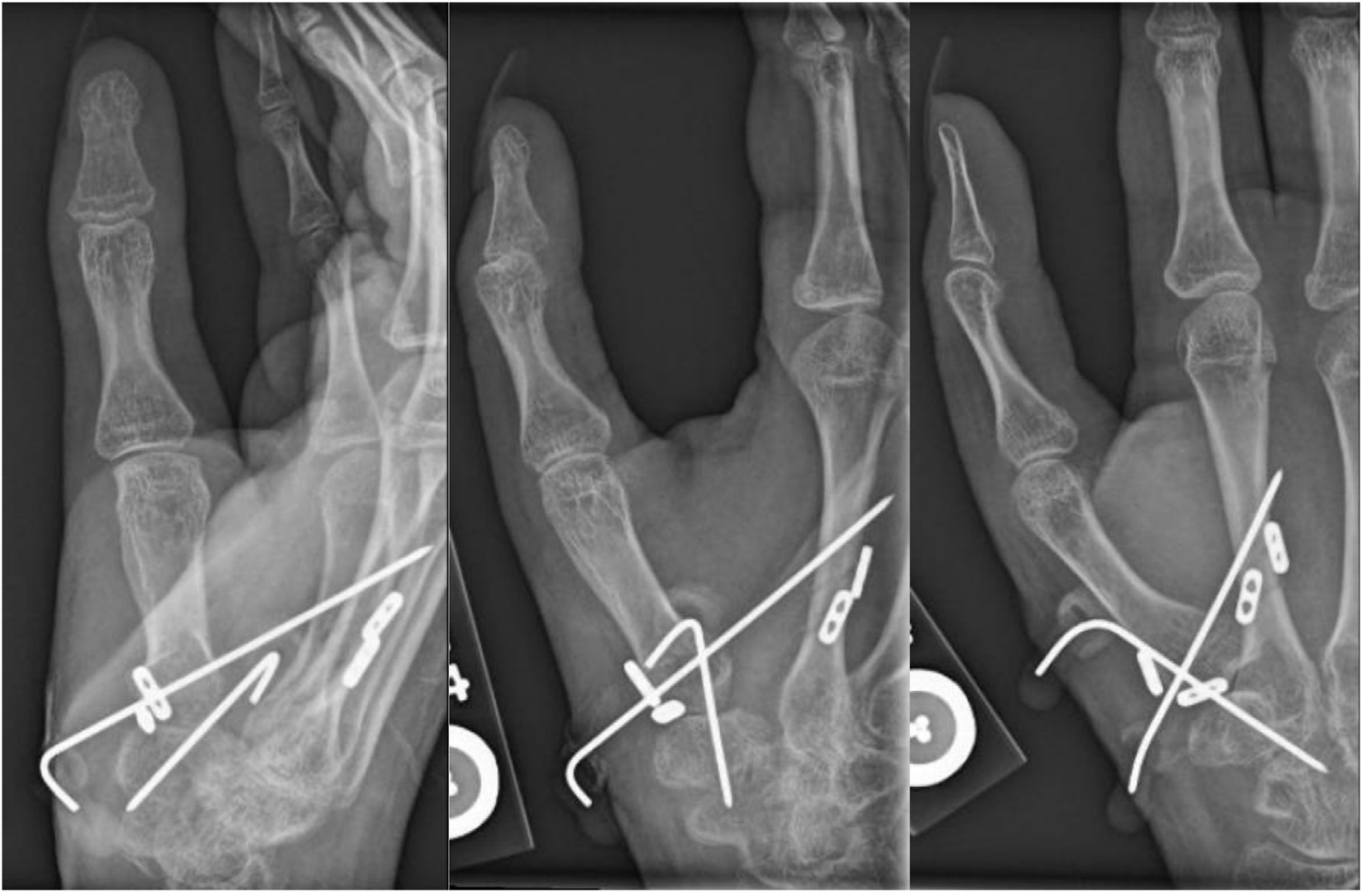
Postoperative day 13 posteroanterior, oblique and lateral right thumb radiographs showing reduction of the thumb CMC joint stabilized with two Arthrex Mini Tightropes and two K-wires.
